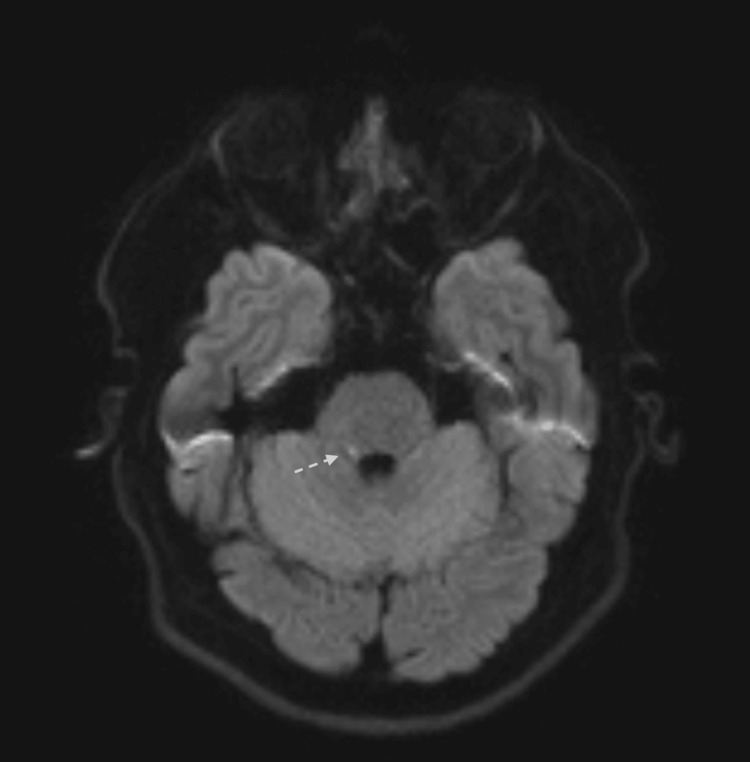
MRI brain DWI view showing small left pontine insult.
